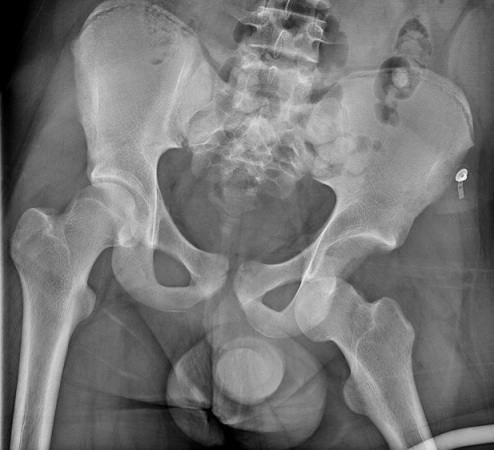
Radiographie montrant la luxation antero-inferieur gauche
